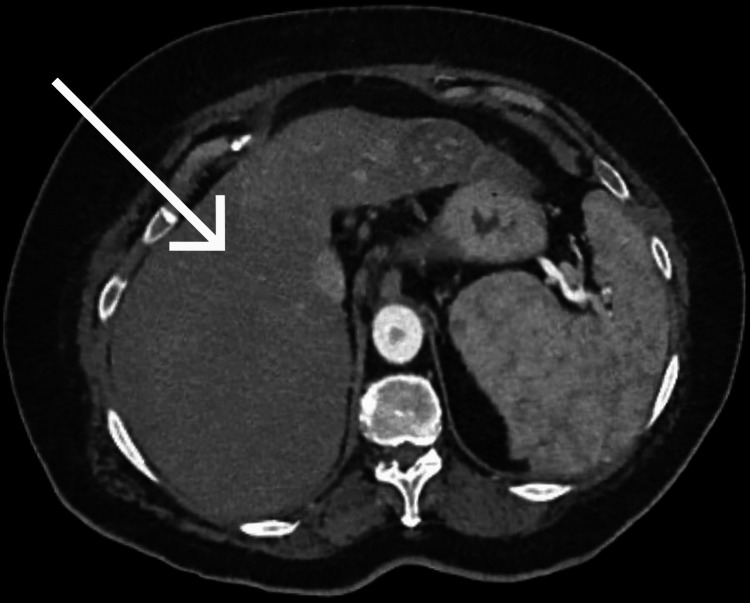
Triphasic CT scan showing a large exophytic heterogeneously enhancing mass centered in the midepigastric region, as marked by the arrow
